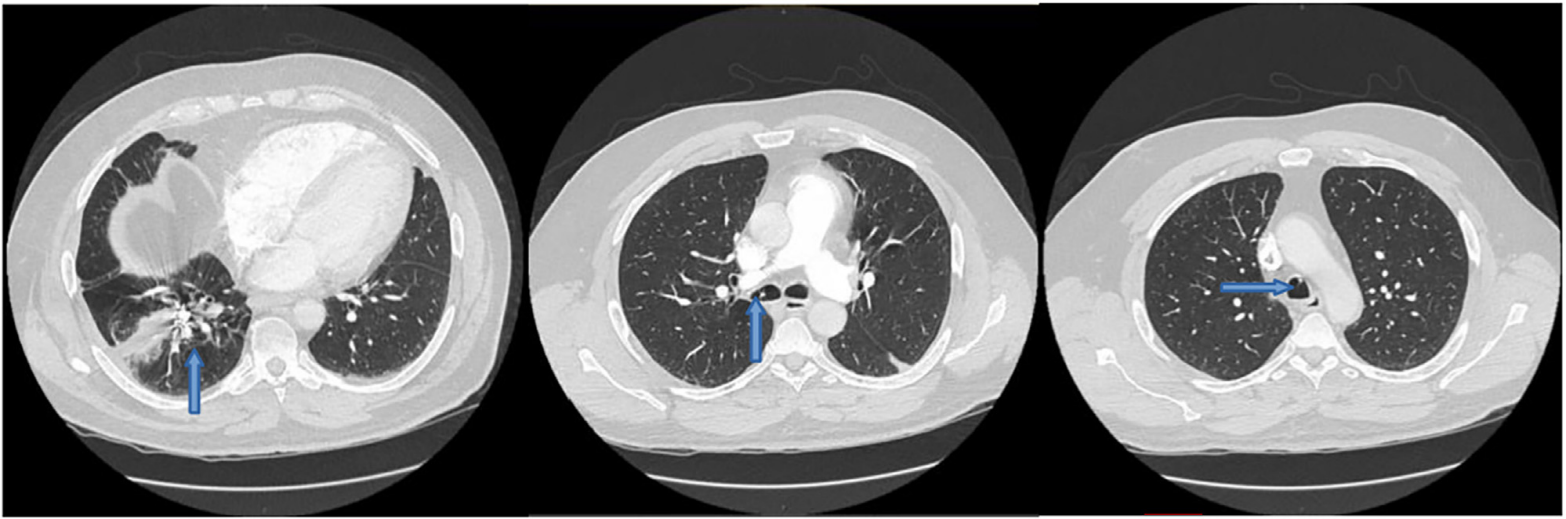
Computed tomography pulmonary angiogram with embolization coils in right lower lobe segmental pulmonary artery with extension into the trachea (arrows).
